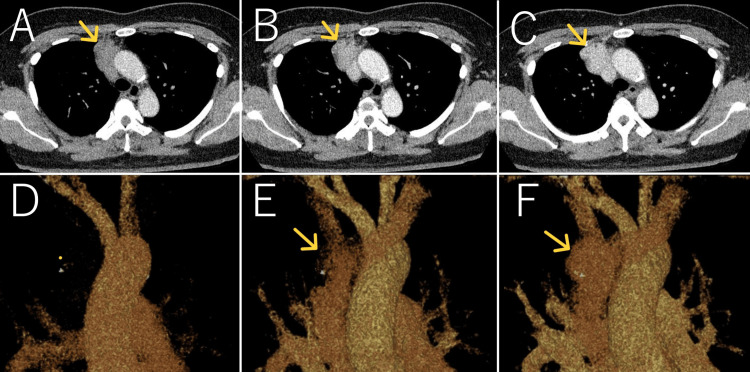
Dynamic contrast-enhanced computed tomography (CT) and 4D-CT (injected via the leg) Dynamic contrast-enhanced computed tomography (CT) and 4D-CT injected via the leg. (A) and (D) are 1 second, (B) and (E) are 12 seconds, (C) and (F) are 16 seconds after starting the scan. The mass exhibited no enhancement in the early phase but showed a gradual increase and persistent enhancement after the contrast material reached the left brachiocephalic vein.
